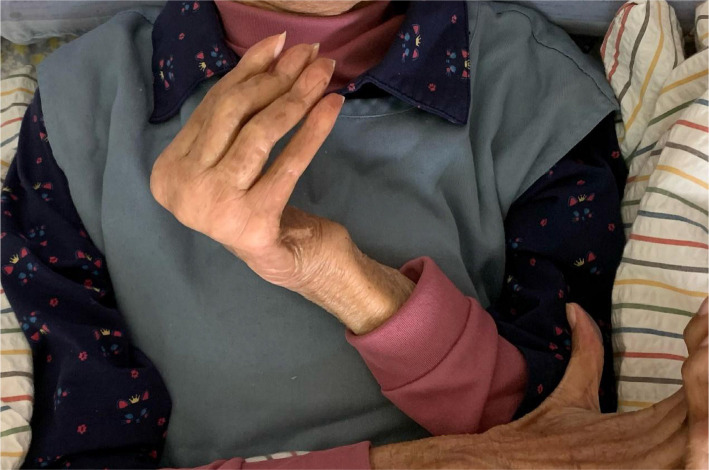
Stage 4 striatal left-hand deformity in a patient with PD for 6 years. Severe bone scintigraphy showed her left metacarpal phalangeal (MCP) at 100° hyperextension, proximal interphalangeal (PIP) at 30° hyperextension, and swan neck deformity of fingers.
